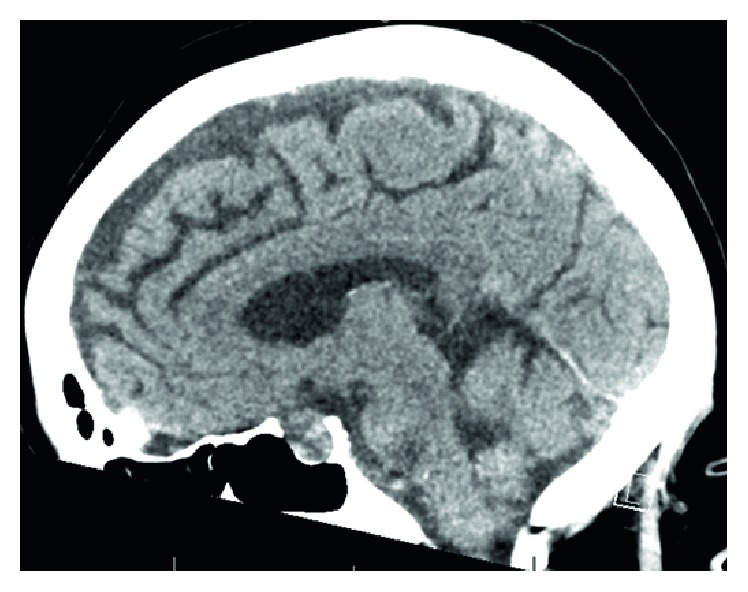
CT head without contrast (sagittal view) showing enlarged sella turcica with increased attenuation suggesting a possible hemorrhage within the tumor.
